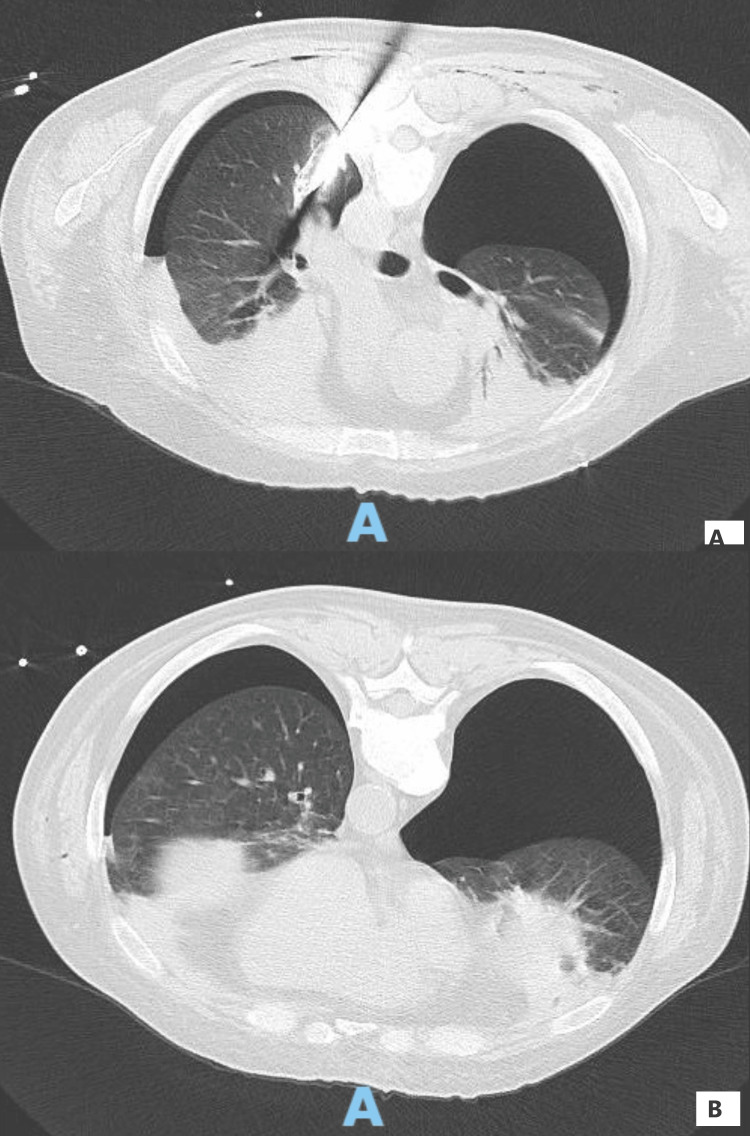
Thoracic CT scan In the first frame, we can see the knife penetrating the lower lobe of the left lung; in the second frame, we can clearly see a large right-sided hemopneumothorax and a moderate left-sided hemopneumothorax. "A" stands for anterior.
